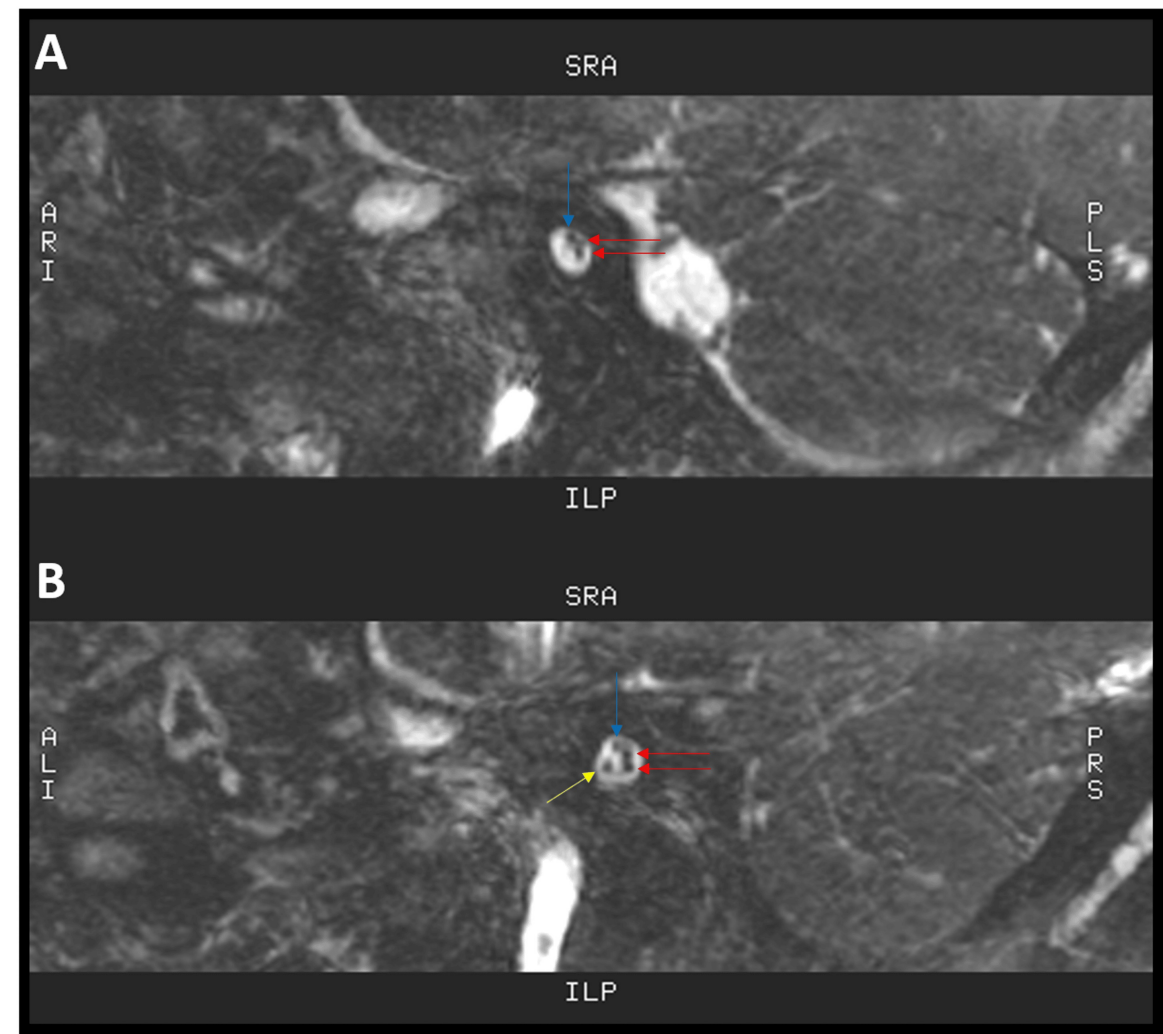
Sagittal oblique reformatted MRI images of the IAC. A) In this image, three nerves are visualized at the fundus of the left IAC: the facial nerve (blue arrow) superiorly, and the superior and inferior vestibular nerves (red arrows) posteriorly. The left cochlear nerve cannot be identified in this image. B) This image demonstrates the four nerves at the fundus of the right IAC: the facial nerve (blue arrow) superiorly, the cochlear nerve (yellow arrow) anteroinferiorly, and the superior and inferior vestibular nerves (red arrows) posteriorly. The letters shown on the MRI images (A, P, R, L, S, I) are orientation markers indicating anterior, posterior, right, left, superior, and inferior directions in the oblique sagittal plane. MRI: magnetic resonance imaging; IAC: internal auditory canal; A: anterior; P: posterior; R: right; L: left; S: superior; I: inferior.
